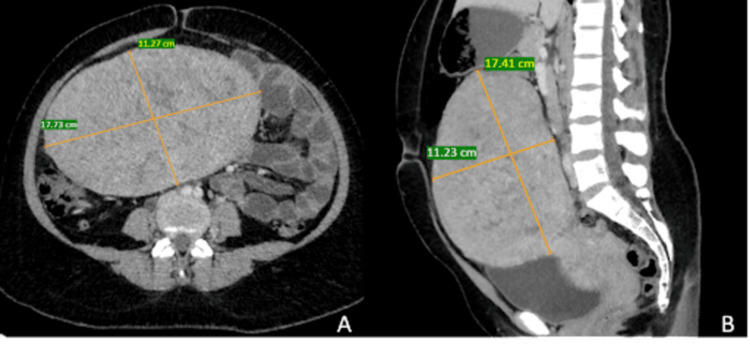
Axial (A) and sagittal (B) sections of contrast-enhanced CT. A large well-defined abdominopelvic solid mass lesion is seen with heterogeneous enhancement. The mass effect in the form of displacement of adjacent bowel loops, mesenteric fat, and omental fat is noted. Craniocaudally, the mass extends from the subgastric region to the uterine fundus.
